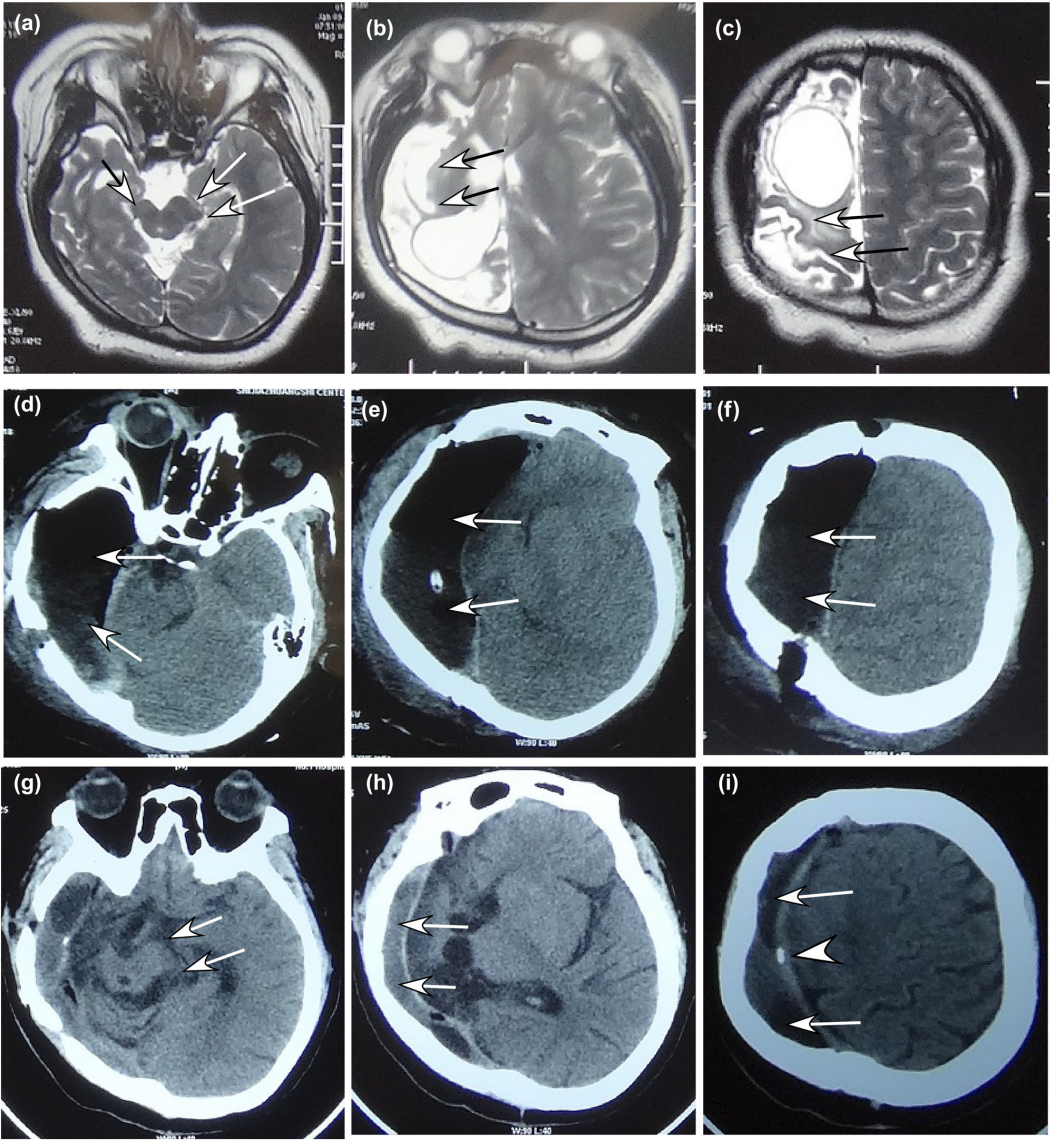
A 21-year-old girl had anatomical hemispherectomy because of cerebral hemorrhage at 1 month after birth and medically intractable epilepsy for 16 years. (a–c) Before the hemispherectomy, magnetic resonance imaging of the brain revealed compensatory enlargement (double arrows in A) of the left cerebral peduncle, atrophic right cerebral peduncle (single arrow in A), and atrophic brain tissue on the right (double arrows in B and C). (d–f) CTS at 8 days posthemispherectomy demonstrated right-shifted midline structures and the residual intracranial space (double arrows) with accumulated air and fluid. (g–i) CTS 3 years later revealed enlarged cerebral peduncle on the left (double arrows in G), further right-shifted midline structures and shrank epidural space (double arrows in H and I) under the thickened skull. The arrow head indicates calcification of the dura mater separating the residual epidural space and the brain tissue on the left.
